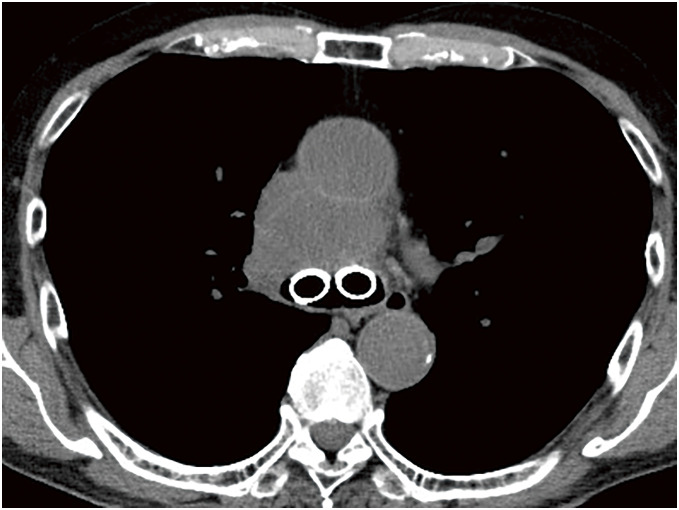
A CT scan taken 65 days after stent placement shows that the tumor has shrunk significantly and the airway stenosis has been resolved. CT, computed tomography
